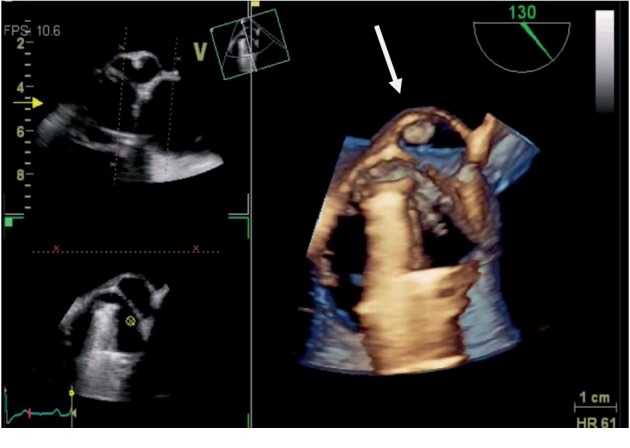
3D reconstruction through transoesophageal echocardiography showing the mitral-aortic intervalvular fibrosa with a pseudoaneurysm (arrow) communicating with the left ventricle outflow tract and a free thrombus inside.
